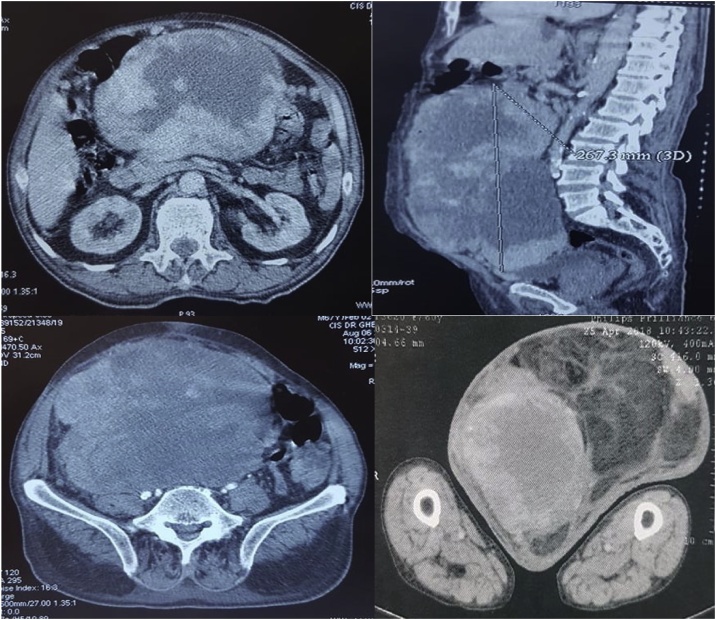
CT scan images revealing a heterogeneously enhancing fatty mass with solid nodules and necrotic areas, with craniocaudal extension of over 40 cm from the retroperitoneum to the inguinoscrotal region, compressing the abdominal aorta and the intestines to the left side of the abdomen.
